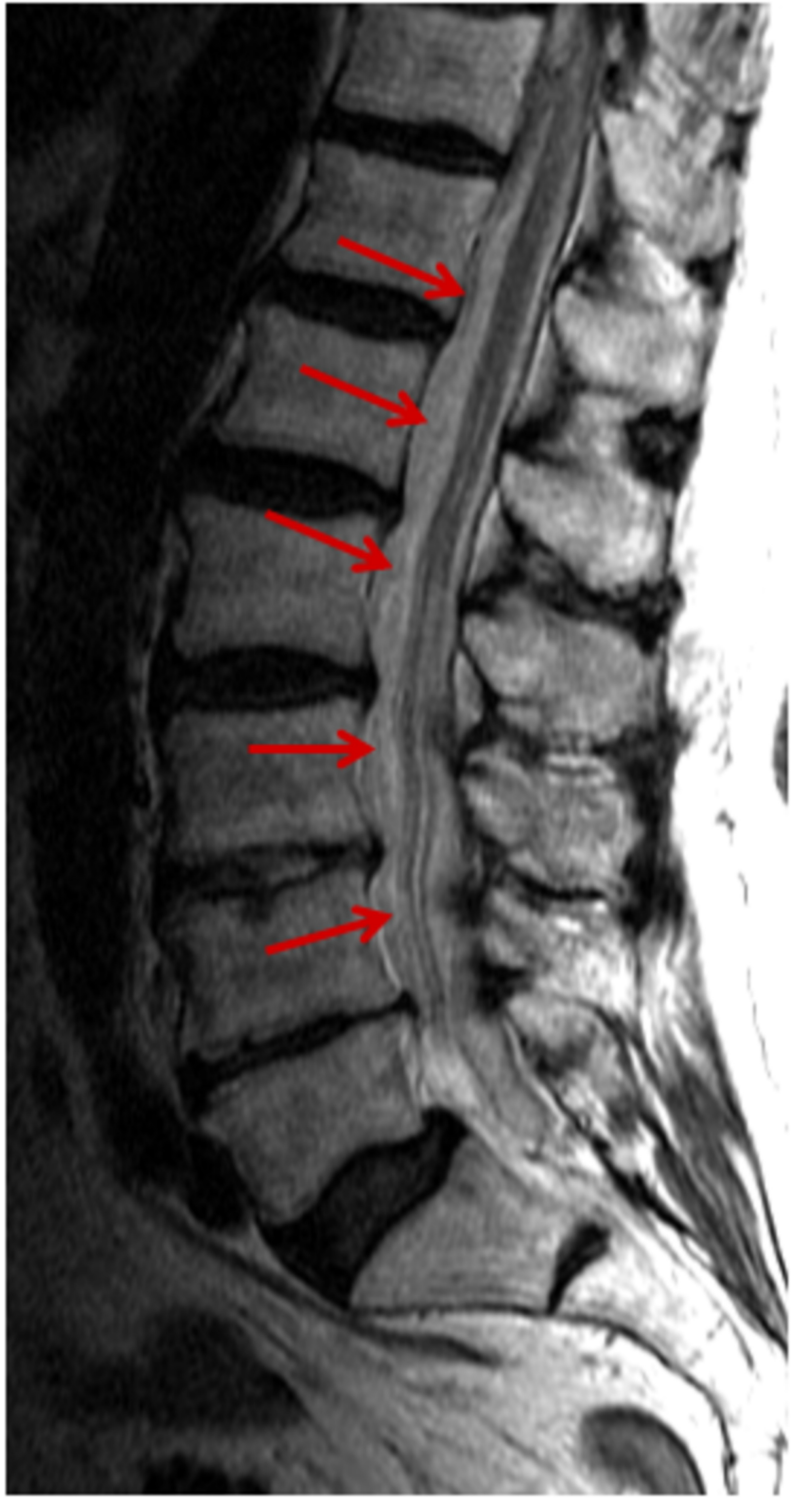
MRI scan, T2-weighted sagittal view demonstrates diffuse subdural intraspinal hematoma throughout the lumbar spine (solid arrows).
